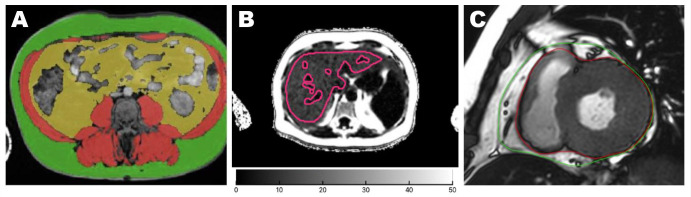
Quantification of adipose tissue from MRI axial images. Areas of abdominal adipose tissues were acquired at the vertebral L3 level. Green: SAT; yellow: VAT (A). Liver PDFF was quantified from the whole liver region of interest marked in red lines on a parametric map, avoiding image artifacts and major vessels (B). EAT was segmented between the epicardium (red line) and the pericardium (green line) on both ventricles at end-systole from the basal to apical short-axis cines. The paracardial fat outside the pericardium was not included in the EAT contour (C). EAT, epicardial adipose tissue; SAT, subcutaneous adipose tissue; VAT, visceral adipose tissue.
